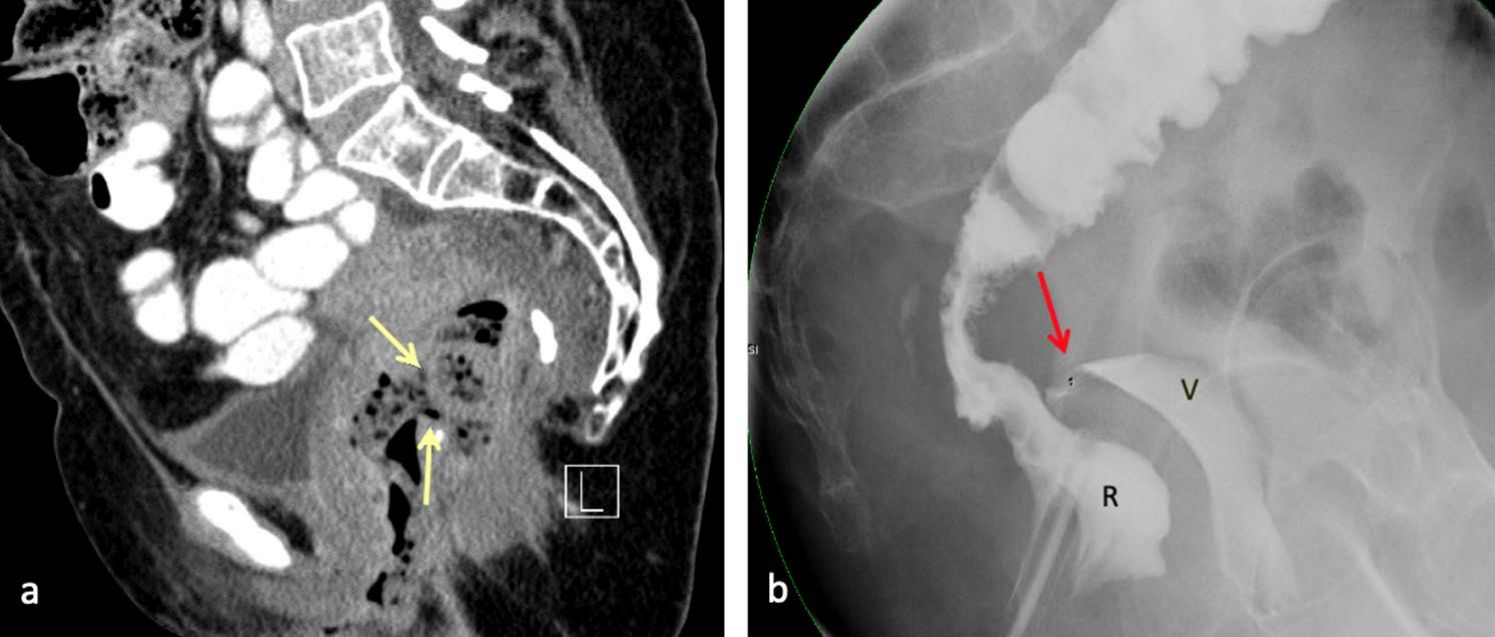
61-year-old female with history of colon cancer status post-radiation and low anterior resection, with subsequent development of radiation-induced rectovaginal fistula. Sagittal contrast-enhanced CT a demonstrates direct communication of the lower rectum anteriorly with the vaginal canal (yellow arrows). Feculent material and air are seen within the vaginal canal and there is loss of the normal fat plane between the vagina and rectum, compatible with a rectovaginal fistula. Gastrograffin enema b demonstrates contrast material filling the rectum (R) and leaking into the vagina (V) through a narrow fistula (red arrow) extending from the upper rectum near the rectosigmoid junction to the vaginal fornix, consistent with rectovaginal fistula
